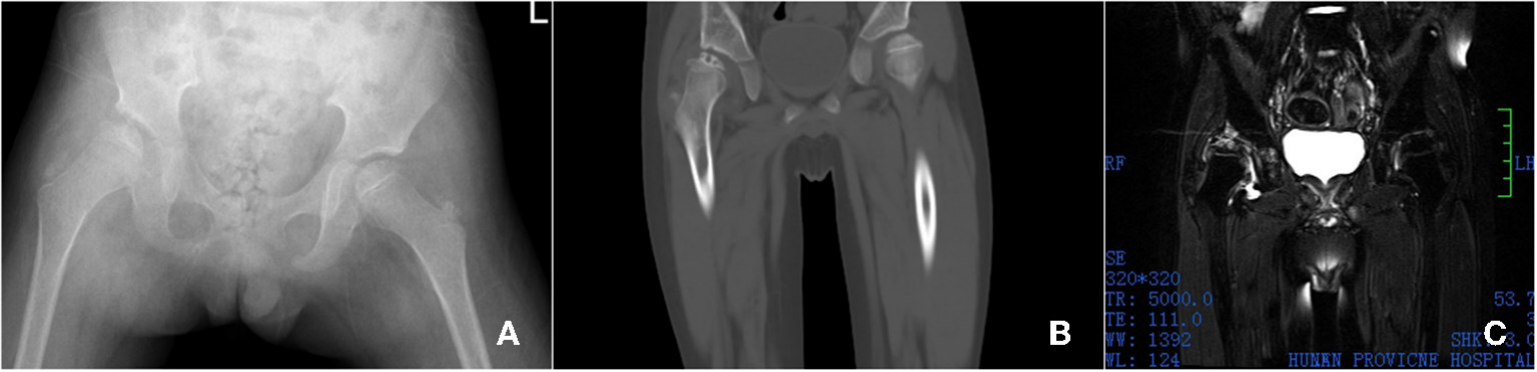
Imaging examination, X-ray shows the right femoral skull epiphysis becomes flat, smaller, irregular in shape, multiple cystic low-density lesions are seen inside, the right acetabulum is flat, and the right hip joint space is widened. The right femoral head shifted outwards and upwards (A). CT shows the right femoral head epiphysis was flattened, several microcystic changes were observed on the upper edge, the right femoral head slightly shifted outwards and upward, and the right acetabulum was slightly flattened (B). MRI showed that the right side Femoral skull epiphysis became flat and local bone collapsed. There were multiple small patchy T2WI hyperintensity shadows under the right femoral metaphysis and right acetabular articular surface (C).
